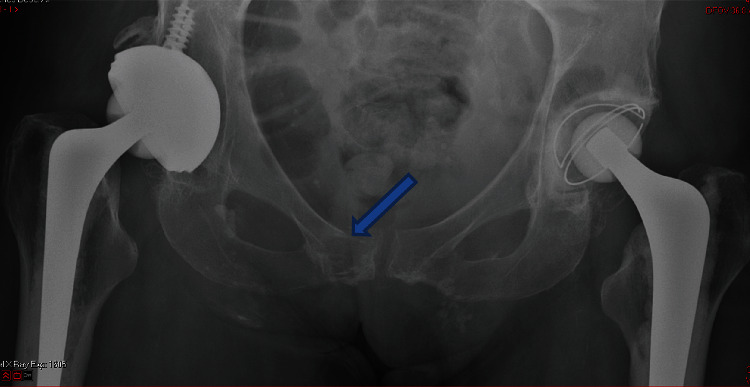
Plain radiograph showing irregularity in the left pubic bone and new bone formation.
